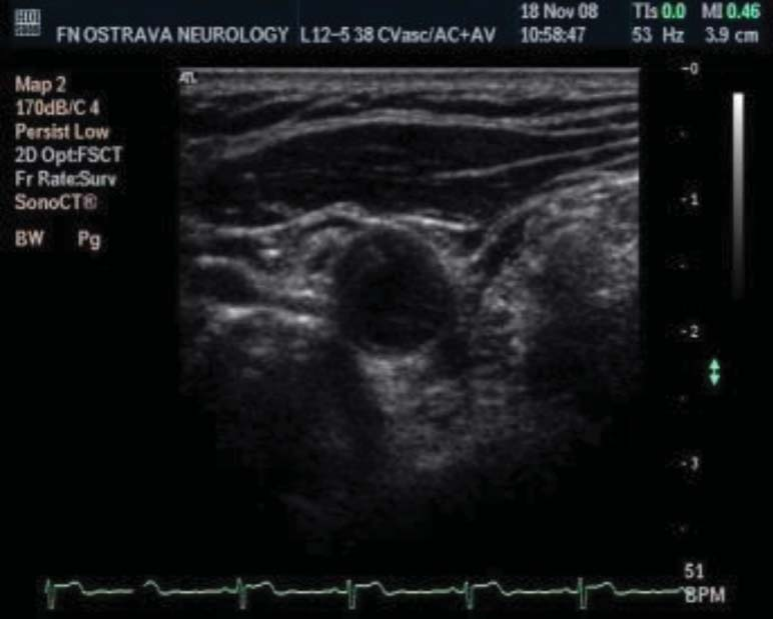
Ultrasonic picture taken from the video signal.
